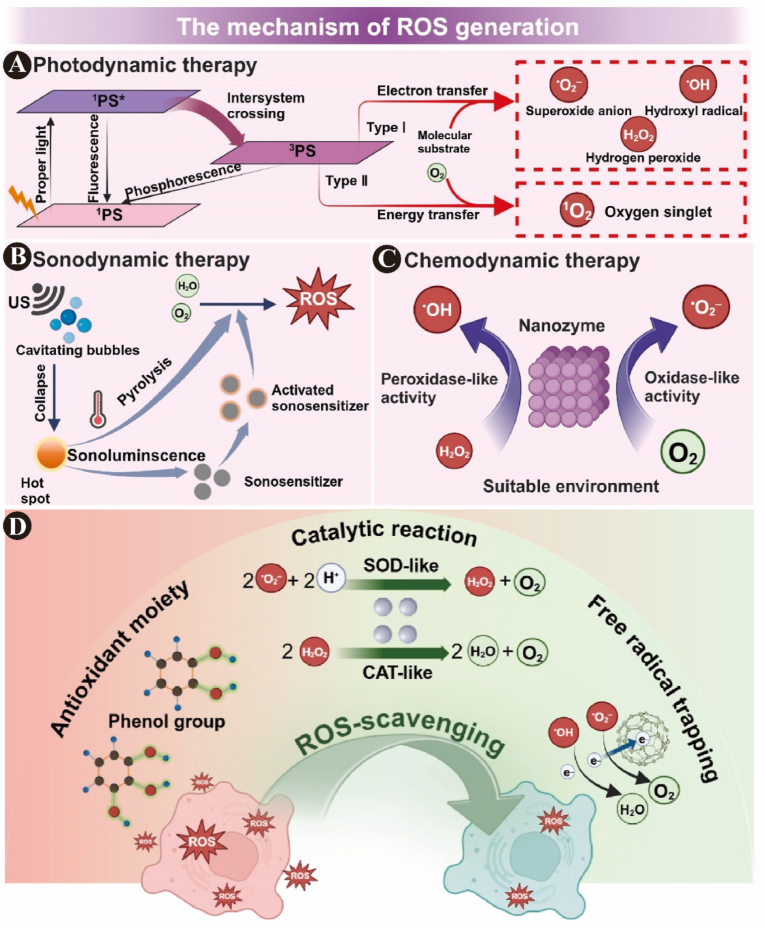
Mechanisms of ROS generation and scavenging. Three major therapeutic strategies for ROS generation: A) PDT produces ROS via light-activated PSs through Type I (electron transfer) or Type II (energy transfer) mechanisms. B) SDT relies on ultrasound-induced cavitation and sonoluminescence to activate sonosensitizers and generate ROS. C) CDT utilizes nanozymes with POD- or oxidase-like activity to catalyze ROS production under specific conditions. D) Strategies for ROS scavenging by nanomaterials, including antioxidant moieties (e.g., phenol groups), enzyme-mimetic catalytic reactions (SOD-like and CAT-like), and free radical trapping, help maintain redox balance and protect against oxidative stress [16]. Reproduced with permission from Ref. [16] Copyright 2024, Elsevier.
